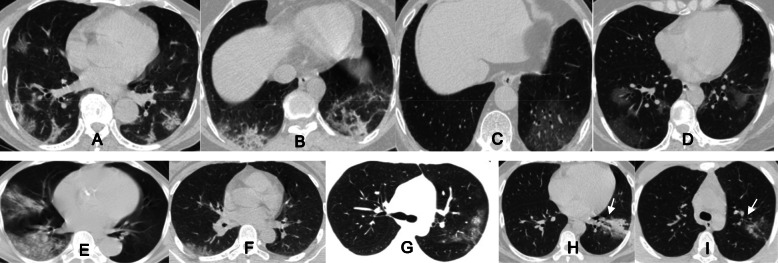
Chest computed tomography (CT) of patients in the 4 familial clusters. a & b The first familial cluster of coronavirus disease 2019 (COVID-19). The chest CT of the husband and wife showed bilateral patchy shadows and grid-like interstitial change in the lower lobes and; c & d The second familial cluster of COVID-19. The chest CT showed a single ground-glass opacity (GGO) in the left lower lung near the pleura of the husband and multiple GGO near pleura in both lungs of the wife. e & f The third familial cluster of COVID-19. The chest CT showed multiple GGOs and consolidation near the pleural of the right lung of the husband and patchy shadows near the pleura in the right lung in the wife. g The son in the third familial cluster had multiple GGOs and patchy shadows in the left lung but the diagnosis could not be confirmed. h & i The fourth familial cluster of *Mycoplasma pneumonia*. Both father and son patients had centrilobular nodules, GGOs, consolidation together with bronchial wall thickening (indicated by arrows)
